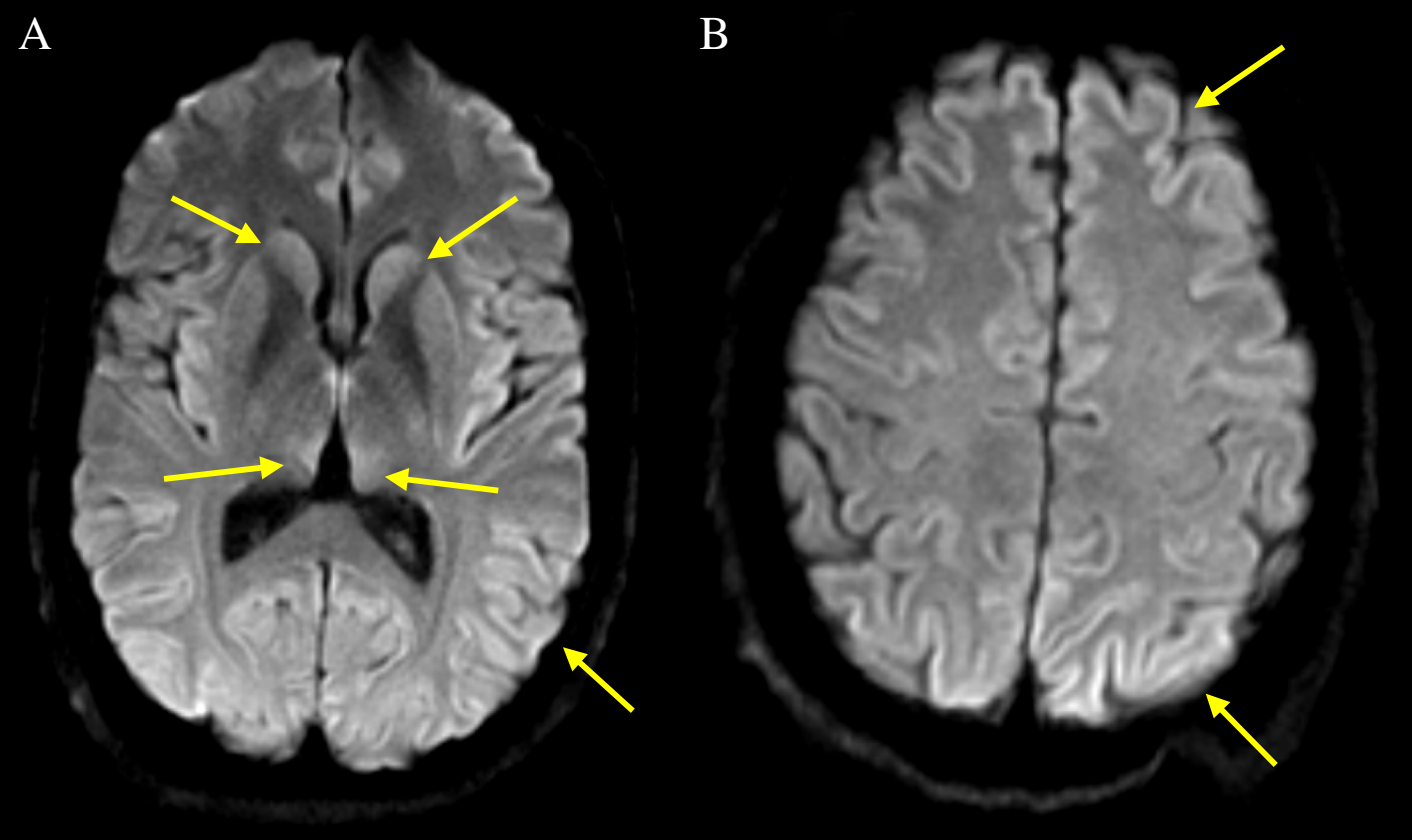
Axial DWI sequences in 'possible’ MV vCJD casedemonstrating (A) basal ganglia and pulvinar hyperintensities (arrows) with associated widespread cortical hyperintensities (A and B)
